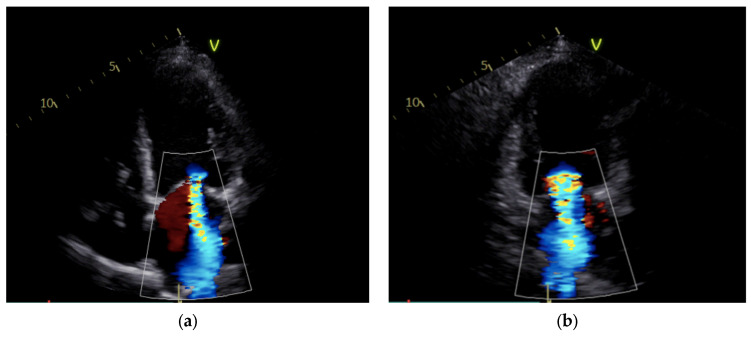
Mitral regurgitation after IABP removal: severe regurgitation is visible in apical 4-chamber view (a) and apical 2-chamber view (b).
